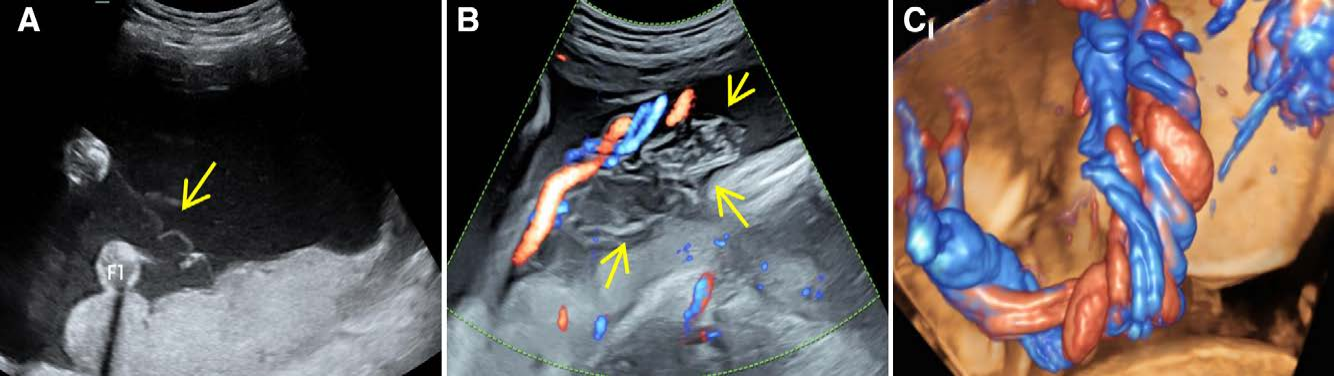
(A) At 26 wk, subtle multilayer folding is evident. (B) At 28 wk, the multilayer folding sign becomes typical.(C) At 31 wk, 3-dimensional color Doppler demonstrates cord entanglement.The yellow arrow points to the multilayer folding sign, representing the ruptured and collapsed dividing membrane.
